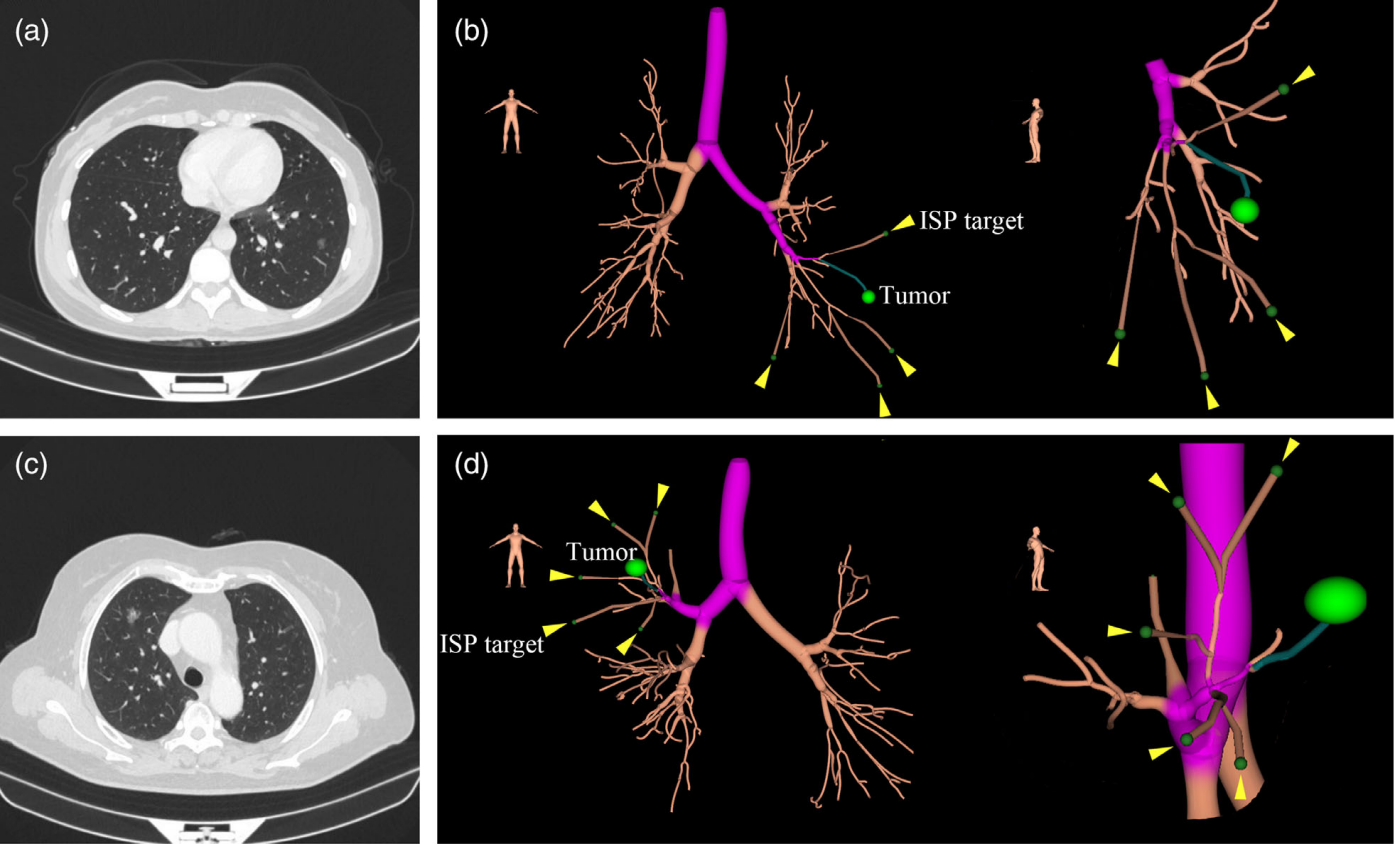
The ENB planning process. (a) CT findings in case 1. (b) The ISP targets* in case 1 between the LS8 and the adjoining segments of LS6/LS9/LS10. (c) CT findings in case 2. (d) The ISP targets in case 2 between the RS3 and the adjoining segments of RS1/RS2. * The ISP targets (yellow arrow) were set at the terminal of the bronchus (approximately 1 cm under the visceral pleura), belonging to the sub‐subsegments of the target segment. CT, computed tomography; ENB, electromagnetic navigation bronchoscopy; ISP, intersegmental plane.
